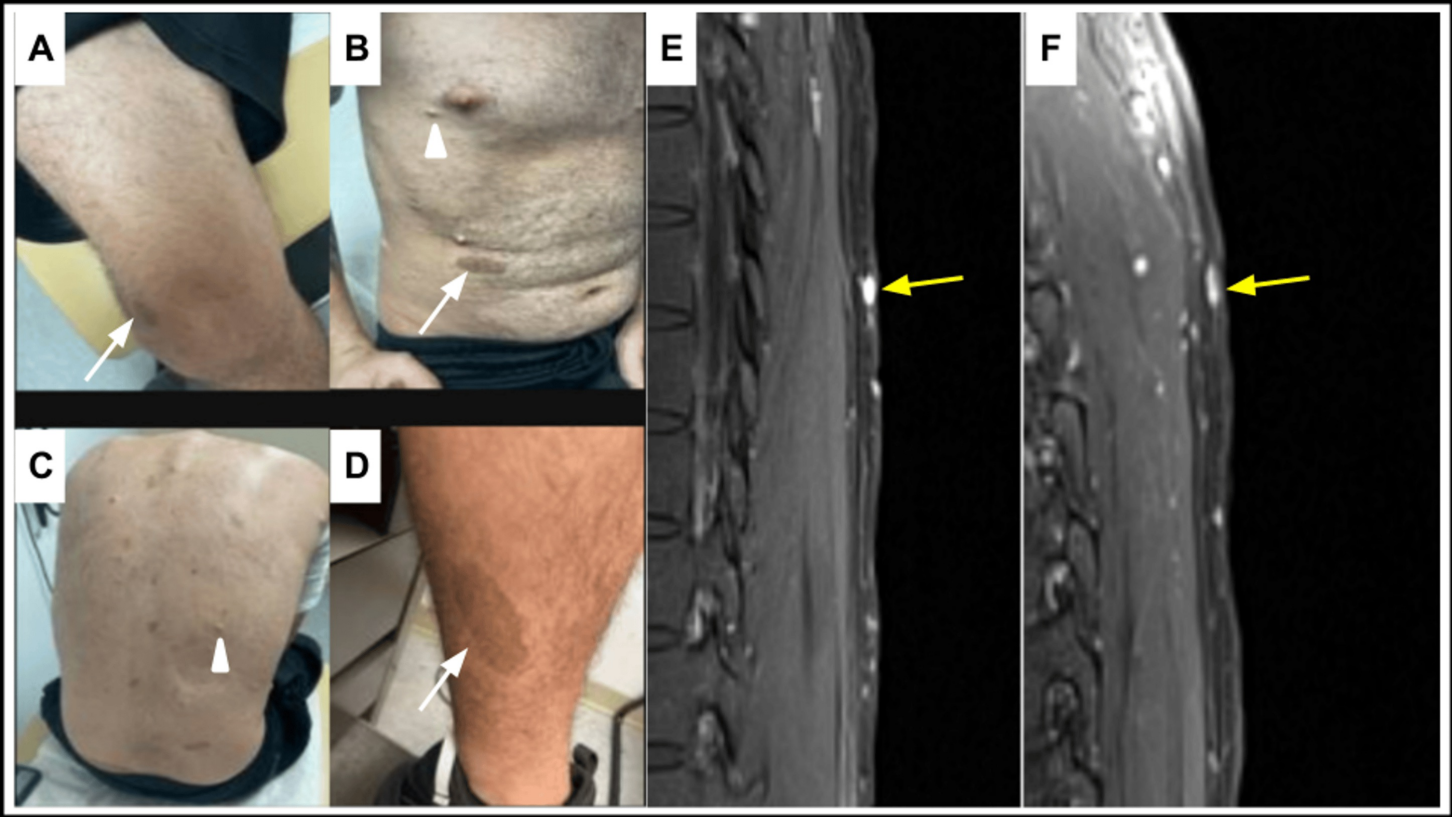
(A-D) Images demonstrate pigmented skin lesions consistent with café-au-lait macules (white arrows). Additionally, there are tiny cutaneous nodules (arrowheads) consistent with cutaneous neurofibromas in this patient. (E) T2-weighted magnetic resonance imaging sequence demonstrates hyperintense subcutaneous lesions (yellow arrow). (F) On gadolinium administration, these lesions demonstrate vivid contrast enhancement (yellow arrow).
